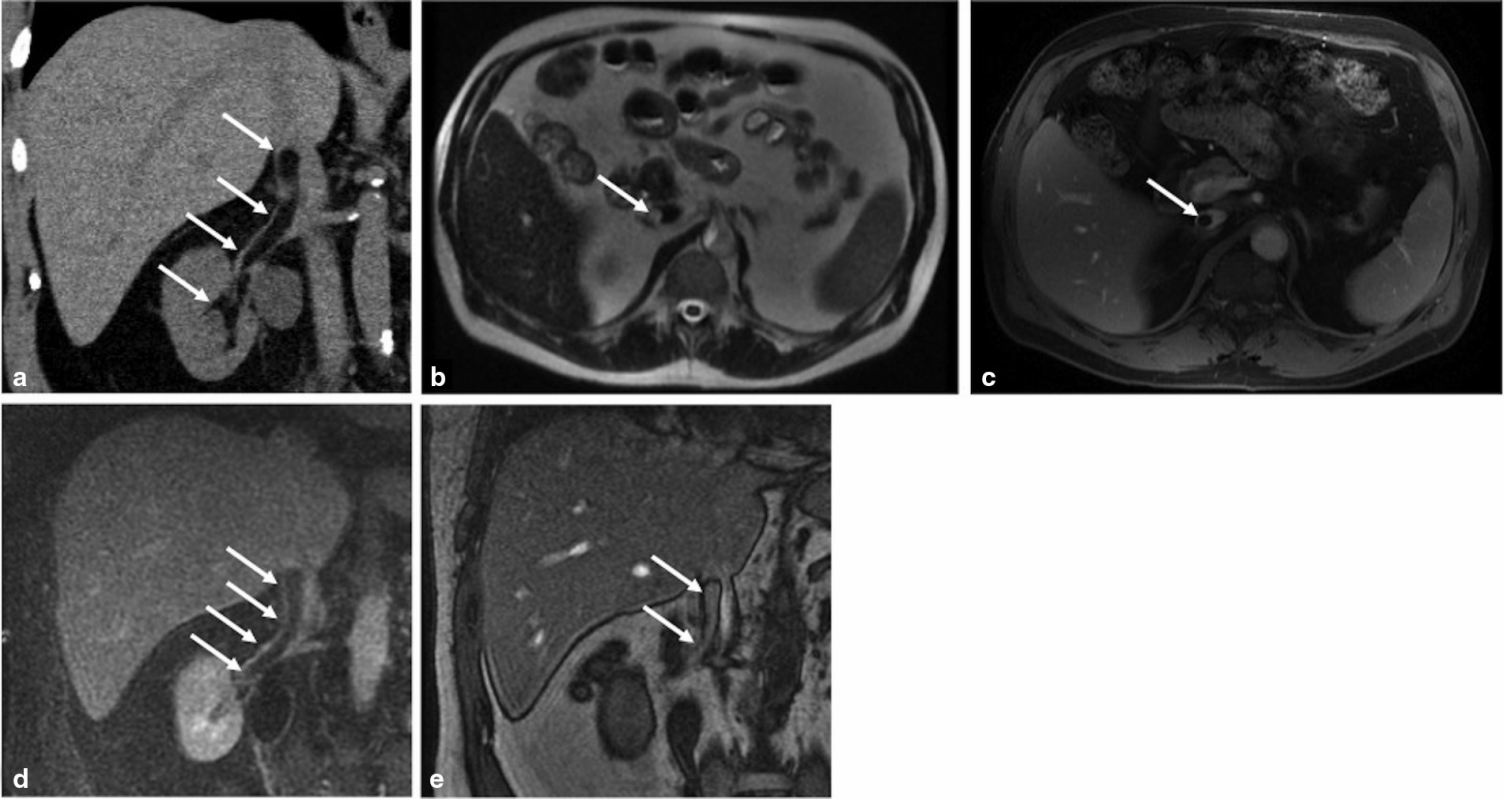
Coronal CT image (a) demonstrates a well-circumscribed homogeneous fat-attenuating intravascular mass (arrows) extending from the renal veins to the inferior vena cava (IVC). Axial *T*_2_ MR image (b) shows a hyperintense round mass in the IVC that subtracts out on post-contrast *T*_1_ fat-saturated axial (c) and coronal (d) MR images as well as demonstrates chemical shift artefact on out-of-phase coronal MRI (e). The lesion is space filling, non-enhancing and nearly all fat, most compatible with an intravascular lipoma.
